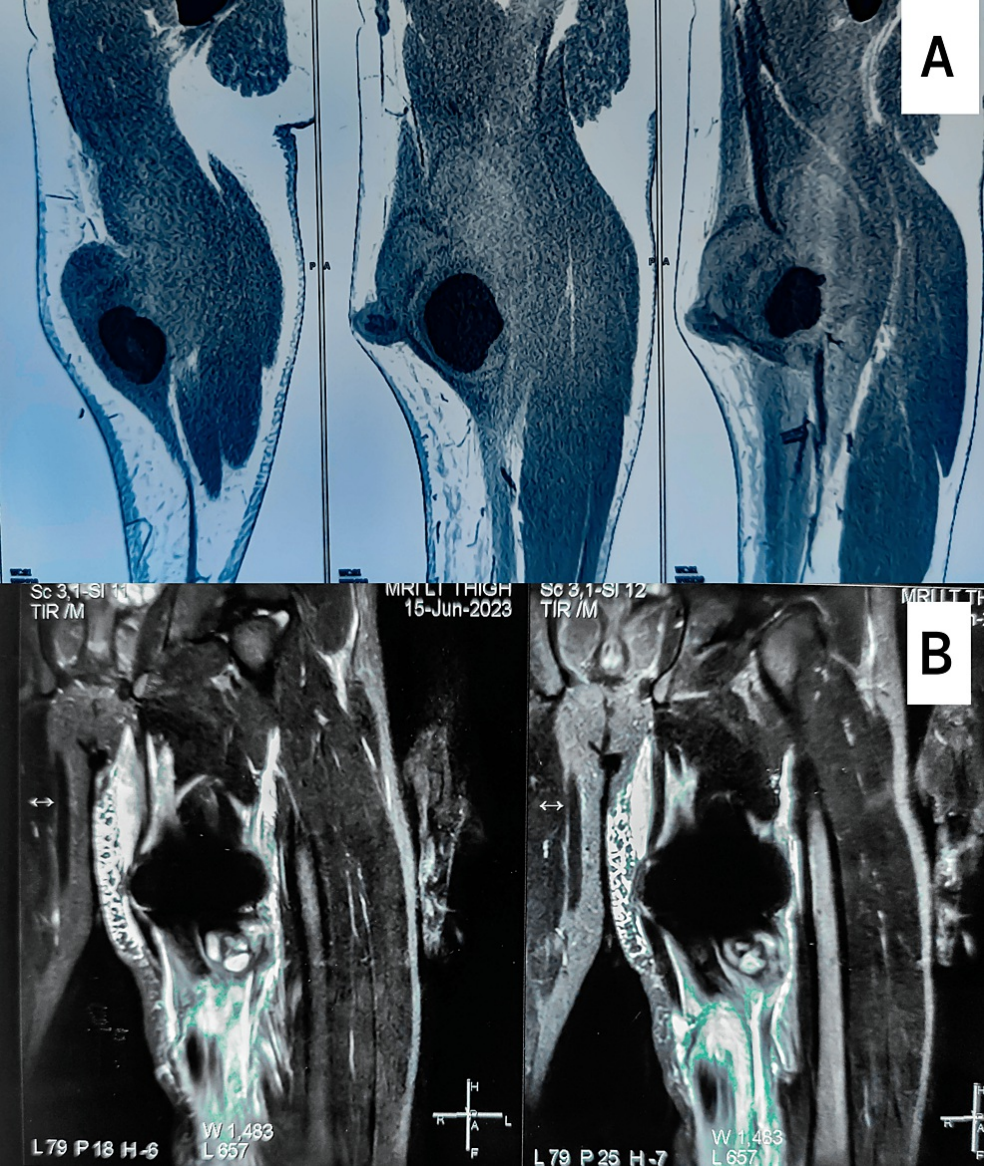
(A) CECT imaging of the left thigh showing well-defined hypodense collection with areas of layered blood attenuation seen in the mid-thigh intramuscular compartment involving the sartorius and adductor longus muscles, measuring 8.4x6.4x14.8 cm in size; (B) MRI of the left thigh showing significant intramuscular injury and hematoma formation along the tract of the foreign body in the adductor longus and adductor magnus muscles, with the foreign body lodged within the hamstring abutment, causing a significant metallic artefact. CECT: contrast-enhanced computed tomography, MRI: magnetic resonance imaging
